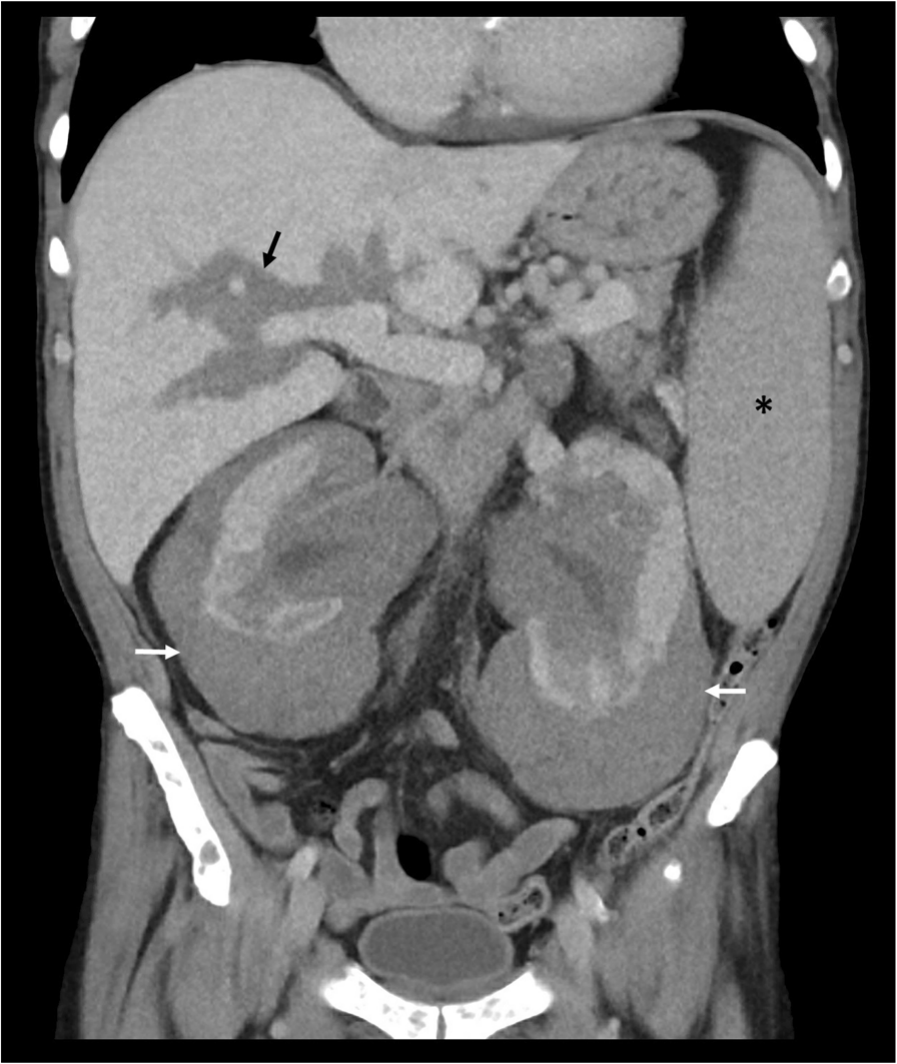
Coronal CT demonstrating bilateral lobular perirenal soft tissue masses, contained by Gerota’s fascia and not causing contour deformity against the kidneys (white arrows), consistent with perirenal extramedullary haematopoiesis, a common site of extramedullary haematopoiesis in the abdomen. Note also the presence of periportal extramedullary haematopoiesis (black arrow) and splenomegaly (asterisk) in addition to osteosclerosis
